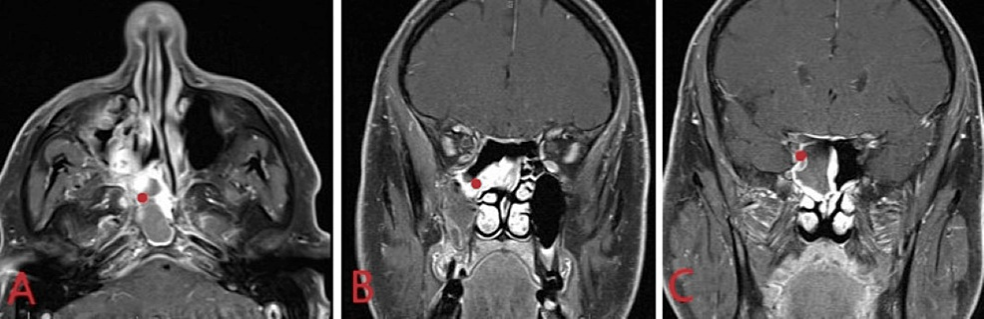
T1 fat-suppressed MRI brain in the axial (A) and coronal (B, C) planes post-induction chemotherapy. Resolution of the mass from the right orbital apex and continued involvement of the right sphenoid sinus laterally and right maxillary sinus anteriorly (red dot).
